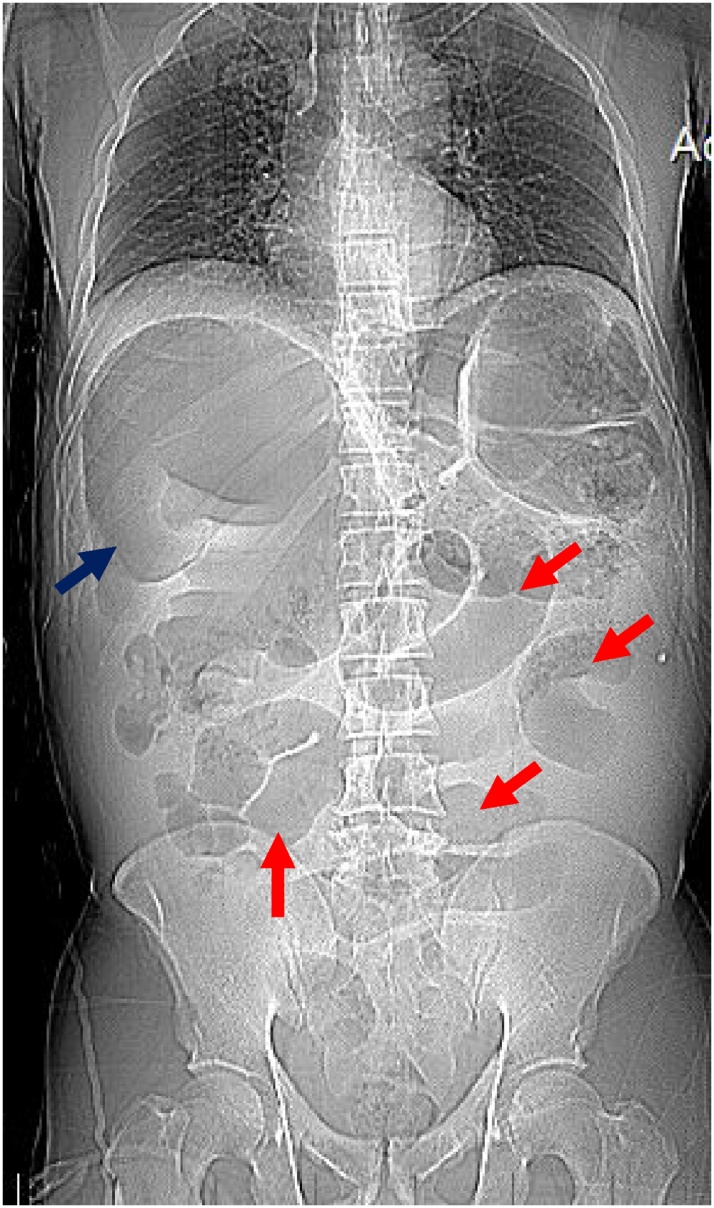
Plain abdominal x-ray in supine position (Red arrows: multiple dilated small bowel loops (jejunum and ileum). Blue arrow: Air fluid level in large bowel loop in the right upper quadrant suspected to be sigmoid colon).
